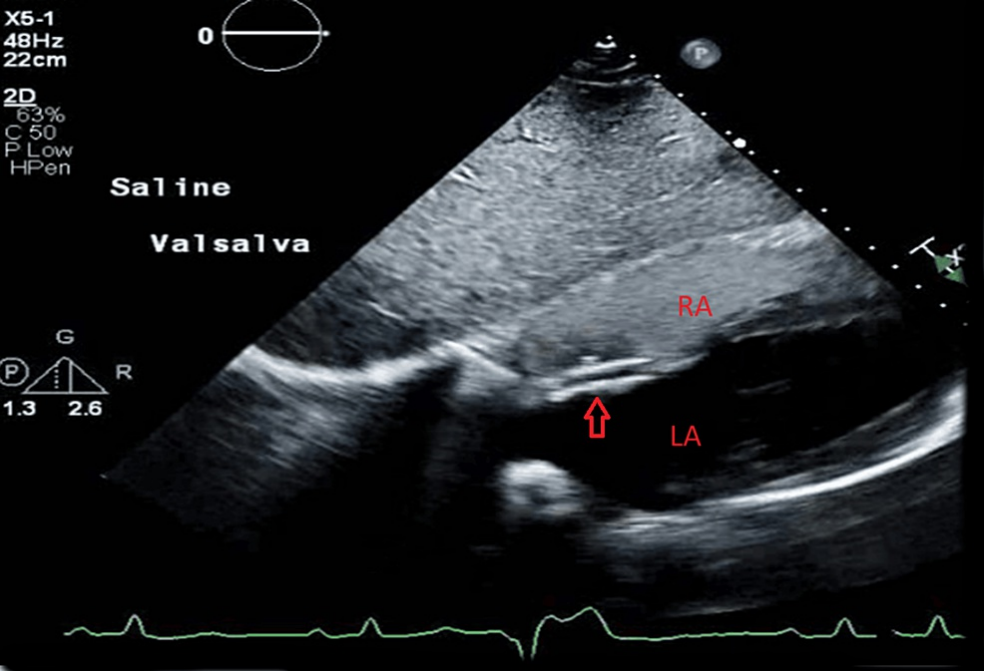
Echocardiography showing closure of the patent foramen ovale. Arrow shows the Amplatzer device in place. Note that there is no passage of bubbles from RA to LA. RA, right atrium; LA, left atrium
